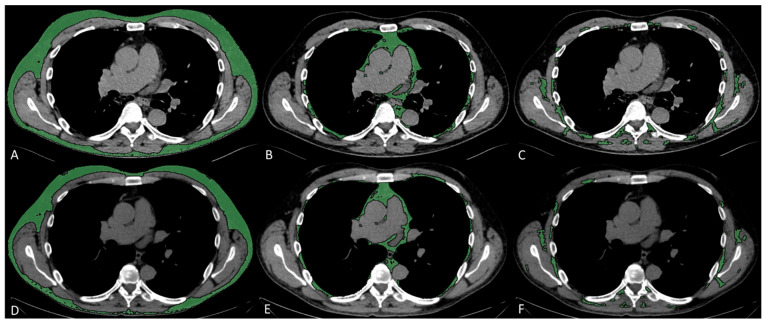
Fat characteristic measurement. Representative CT images analyzed through semiautomated segmentation of different fat compartments. The measurement of baseline SAT (A), VAT (B), and IMAT (C), and 2–3-month follow-up SAT (D), VAT (E), and IMAT (F), showed a decrease in SAT area from 127 to 107 cm^2^ and an increase in SAT density from −104 to −90 HU, a decrease in VAT area from 36 to 31 cm^2^ and an increase in VAT density from −89 to −80 HU, a decrease in IMAT area from 21 to 13 cm^2^, and an increase in IMAT density from −73 to −61 HU.
